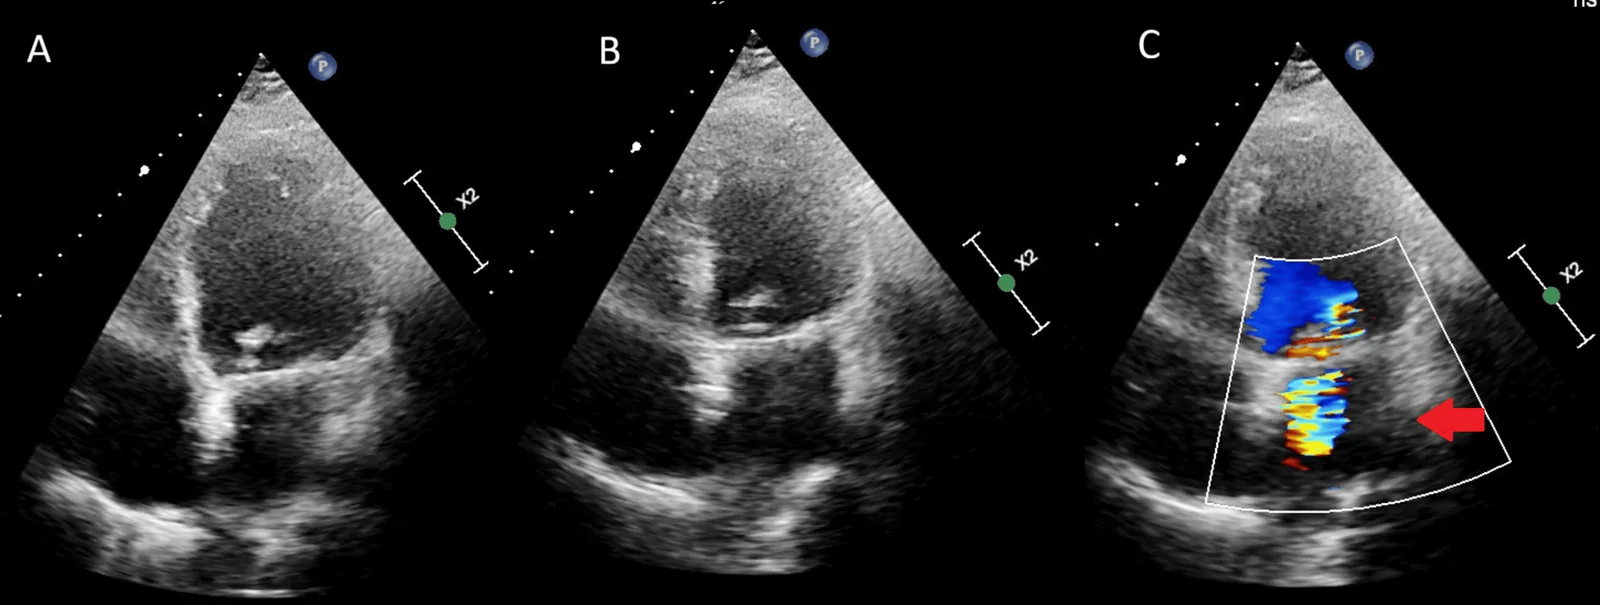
Representative still frames from transthoracic echocardiography. Global left ventricular contractility was assessed using the complete dynamic cine study, which demonstrated mild systolic impairment with a left ventricular ejection fraction of 45–50%. (A–B) Representative grayscale echocardiographic views from the study. (C) Color Doppler demonstrating moderate residual mitral regurgitation, with the regurgitant jet directed into the left atrium (arrow).
